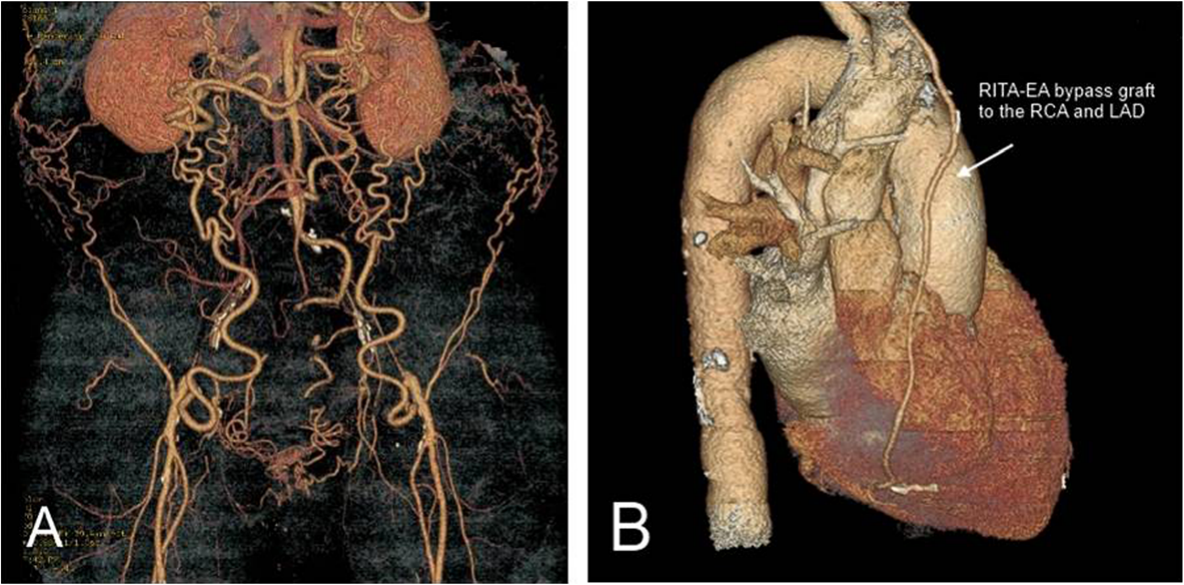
The preoperative and postoperative 3D reconstruction from CT scan.A) A computed tomography image showing total occlusion of the distal portion of the aorta with collaterals to the lower extremities, B) A postoperative cardiac computed tomography demonstrated patency of the sequential RITA – IEA bypass graft to the RCA and proximal LAD.
